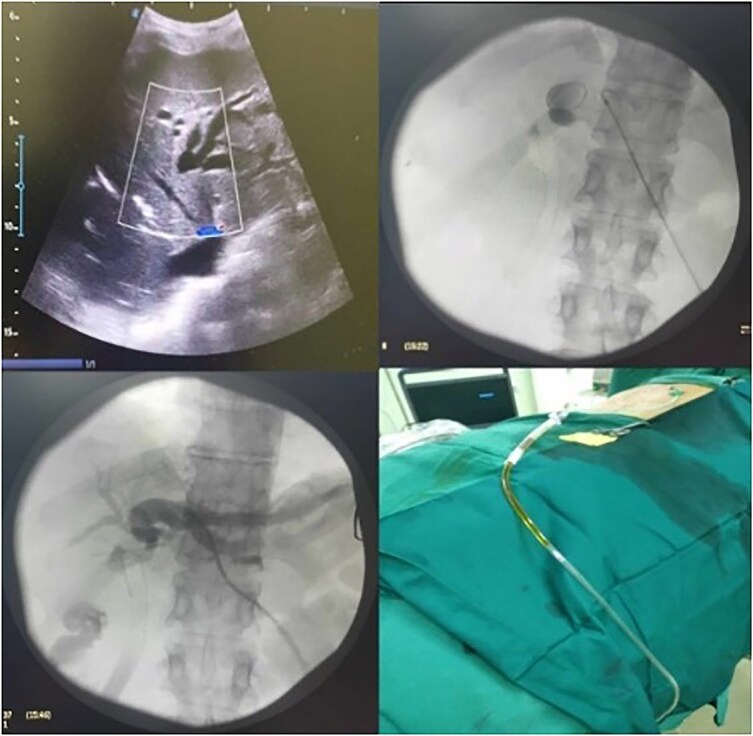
Ultrasound exploration and PTBD placement.
